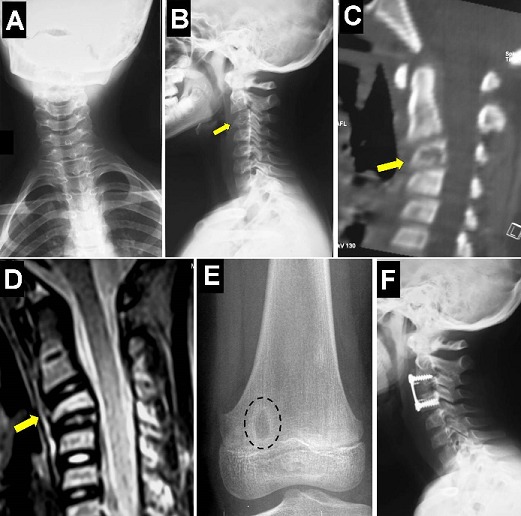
Cervical spine X-rays showing a cervical scoliosis with loss of the normal cervical lordosis and partial collapse of C3 vertebral body (A and B). Sagittal reformatted CT-scan and sagittal T2-weighted MR imaging demonstrating the C3 vertebral corpus destruction (vertebra plana) (arrows) without discitis or spinal cord compression (C and D). Simple left knee X-ray (anteroposterior view) revealing a well-defined solitary metaphyseal lytic lesion in the medial femoral condyle (E). Cervical spine X-ray (lateral view) showing the normal cervical lordosis and the anterior fixation plate (F)
